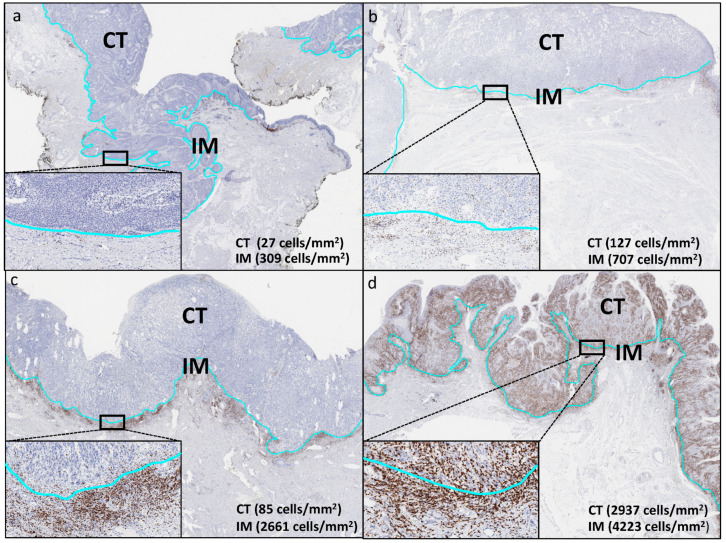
T-cell infiltration patterns in vulvar cancer. Representative images of an immune desert (a); immune excluded (b,c), and an immune inflamed phenotype (d) are depicted. The light-blue line indicates the invasive margin (IM) that represents the outer edge of the center of the tumor (CT). The images are taken at 5× magnification.
